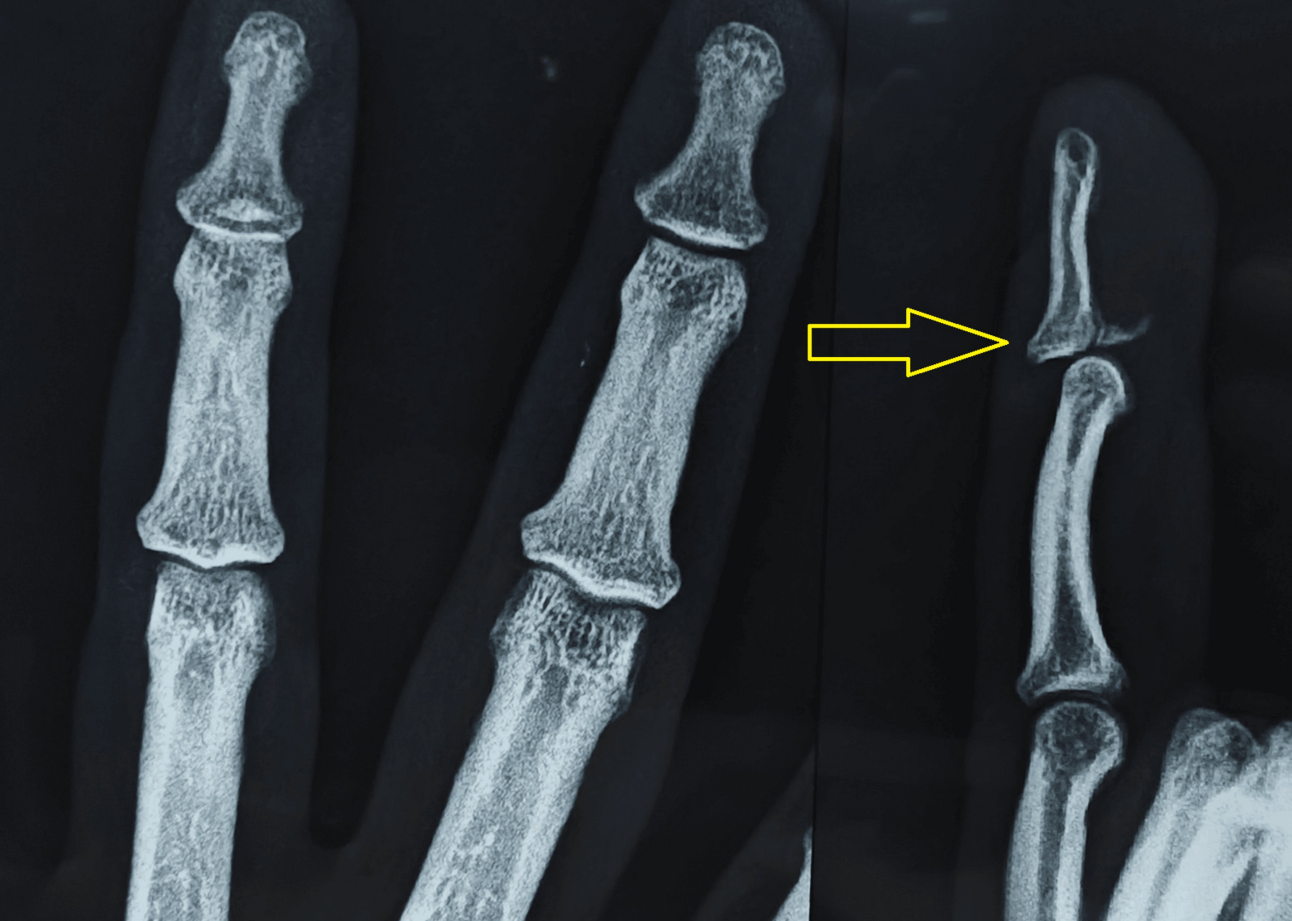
Preoperative radiograph displaying a displaced DIP joint on the lateral view (yellow arrow) DIP, distal interphalangeal
